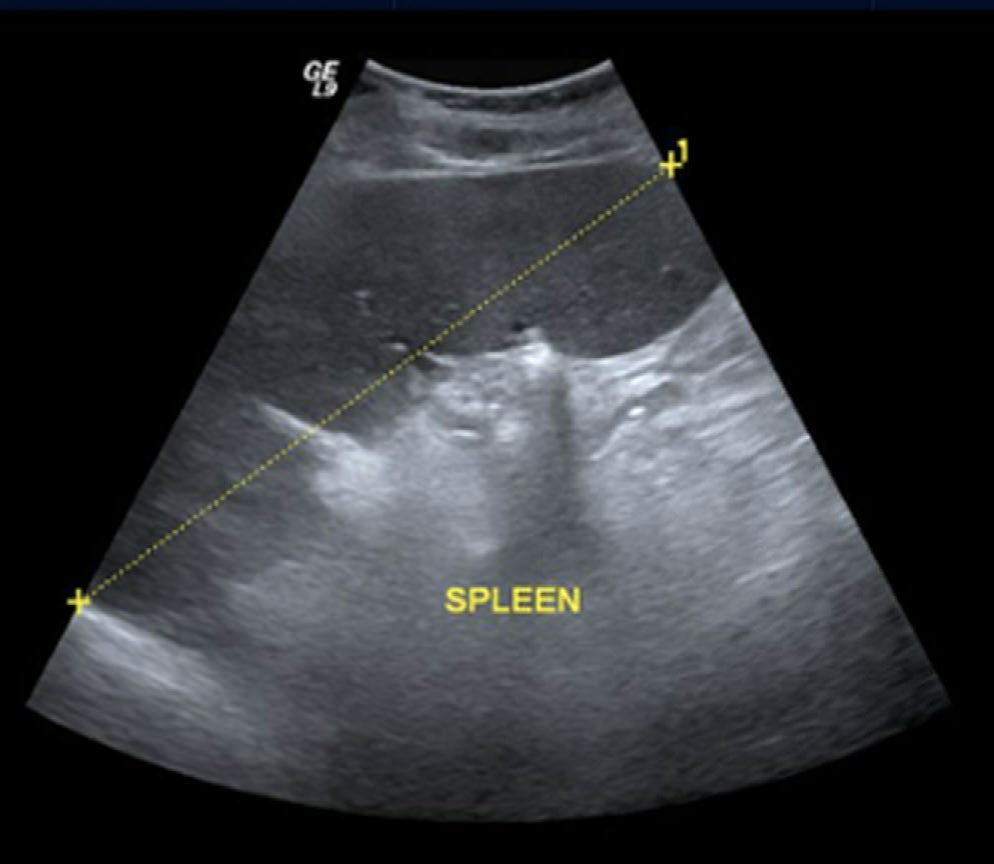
Ultrasound abdomen of Patient 1 showing massive splenomegaly
